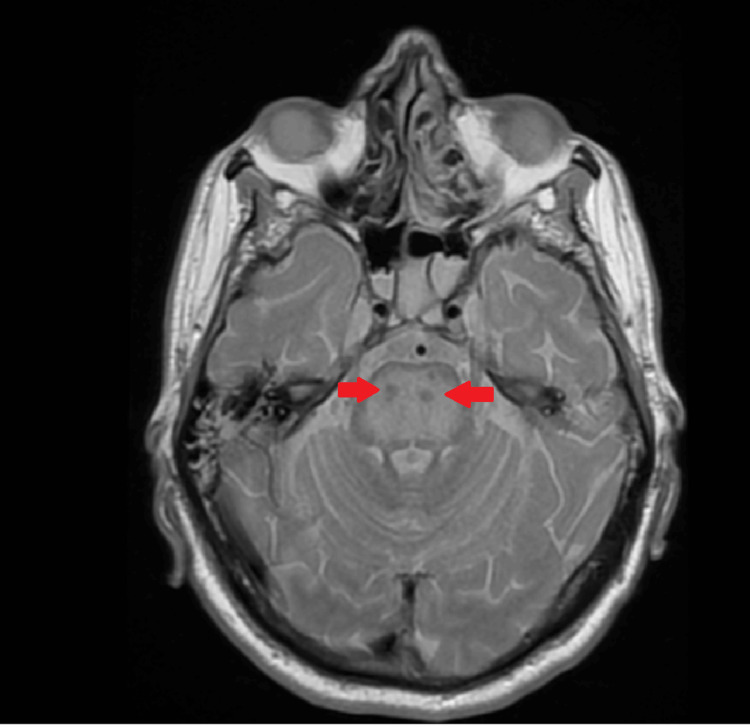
MRI T1 axial image demonstrating low signal in the pons (red arrows)
